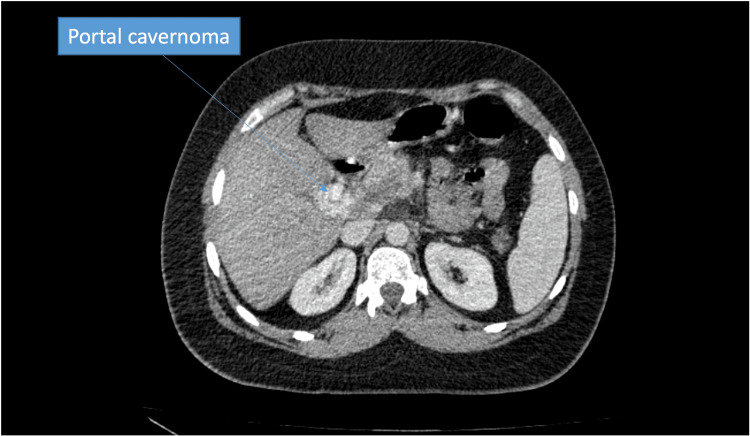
CT angiography showing a cavernomatous transformation of the portal vein.
